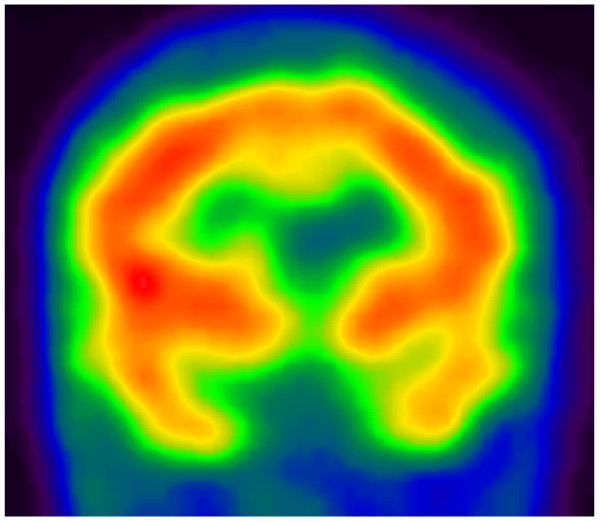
Post-treatment brain perfusion single-photon-emission computed tomography, showing improvement in perfusion in the left temporal lobe.
